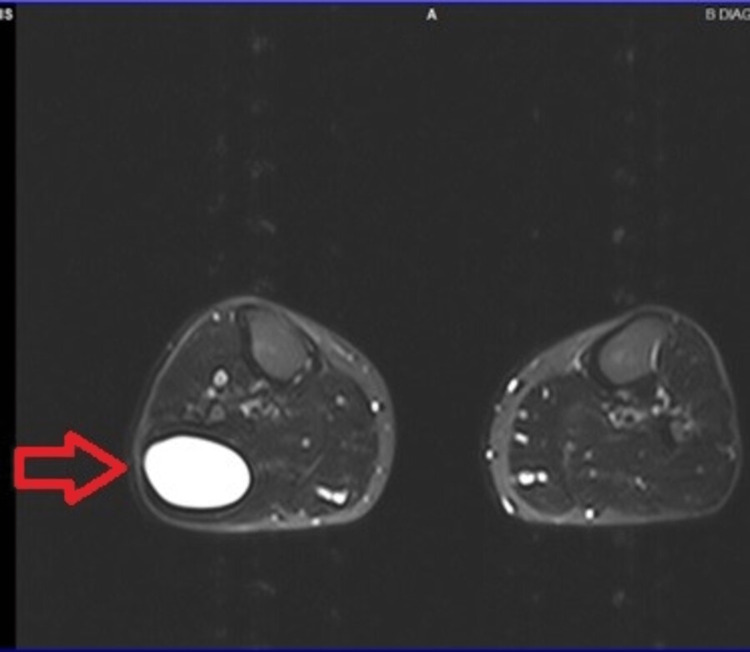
Axial T2-weighted MRI indicates the lesion (red arrow) to the posterolateral aspect of the PTF joint, with cystic form and clear boundaries. MRI: magnetic resonance imaging, PTF: proximal tibiofibular
